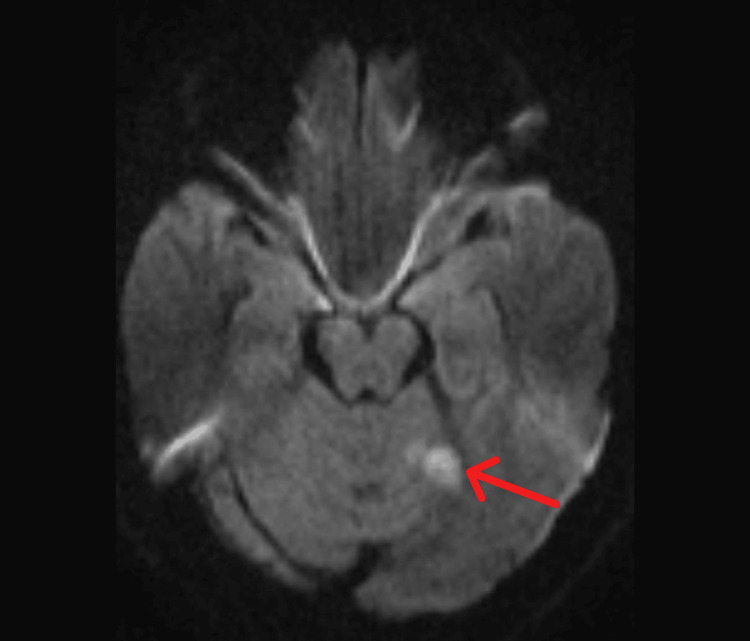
Axial FLAIR at the level of the inferior collicular region showing a patchy area of FLAIR hyperintensity in the left superior cerebellum FLAIR: fluid-attenuated inversion recovery
